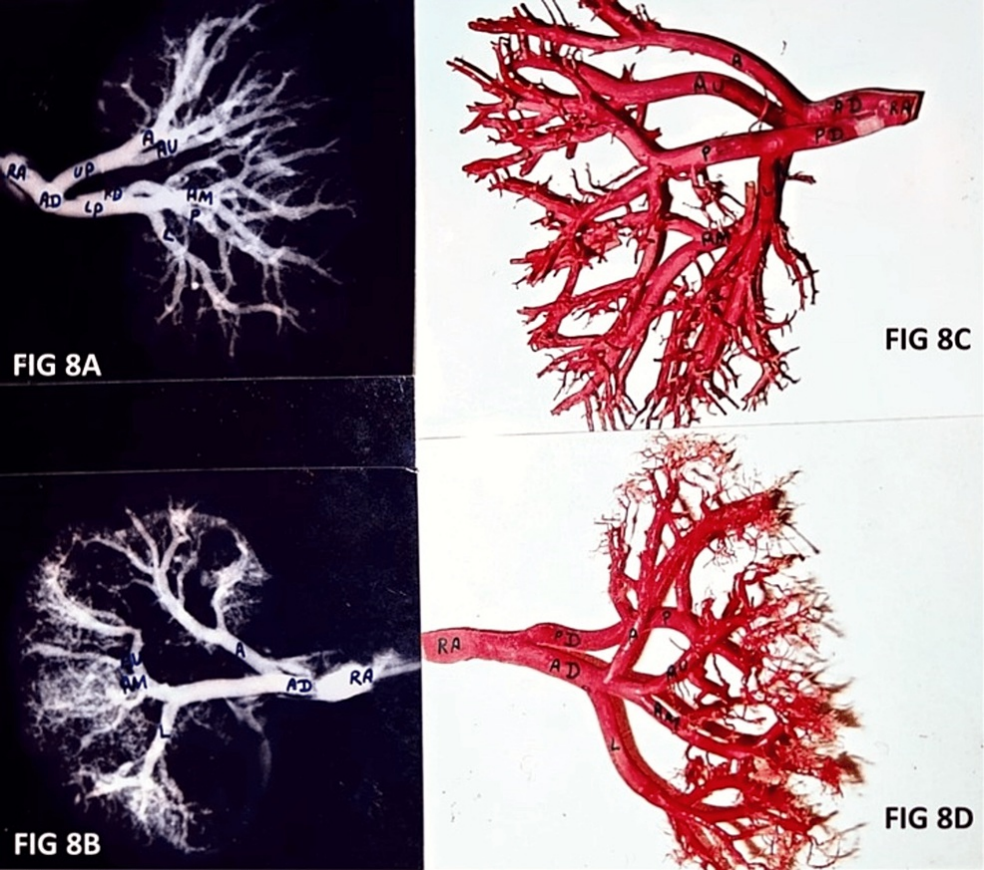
Radiographic images and resin casts showing pattern of different segmental arteries. RA - Renal artery, AD - Anterior division of renal artery, PD - Posterior division of renal artery (A) Radiographic image showing segmental pattern of left renal artery seen in AP view. (B) Radiographic image showing segmental pattern of right renal artery seen in AP view. (C) Resin cast image showing segmental pattern of left renal artery seen from posterior aspect. (D) Resin cast image showing segmental pattern of left renal artery seen from anterior aspect.
